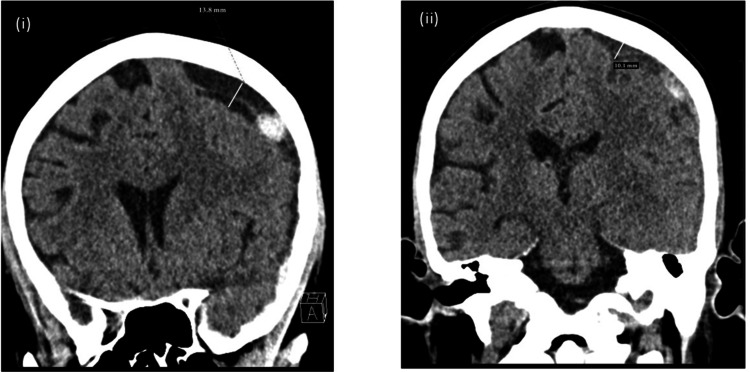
Pre and post-transplant imaging (i) Pre-transplant: CT brain shows a left hemispheric subdural collection with mixed fluid and hyperdense hemorrhagic contents, measuring up to 14 mm in thickness, extending to the left posterior parafalcine region and overlying the left tentorial leaflet, with a slight mild left uncal medialization and up to a 3 mm left-to-right midline shift. (ii) Post-transplant (Day 0): CT brain shows an evolving left hemispheric subdural hematoma with slightly decreased density areas, measuring approximately 10 mm in width and a midline shift of 2 mm.
